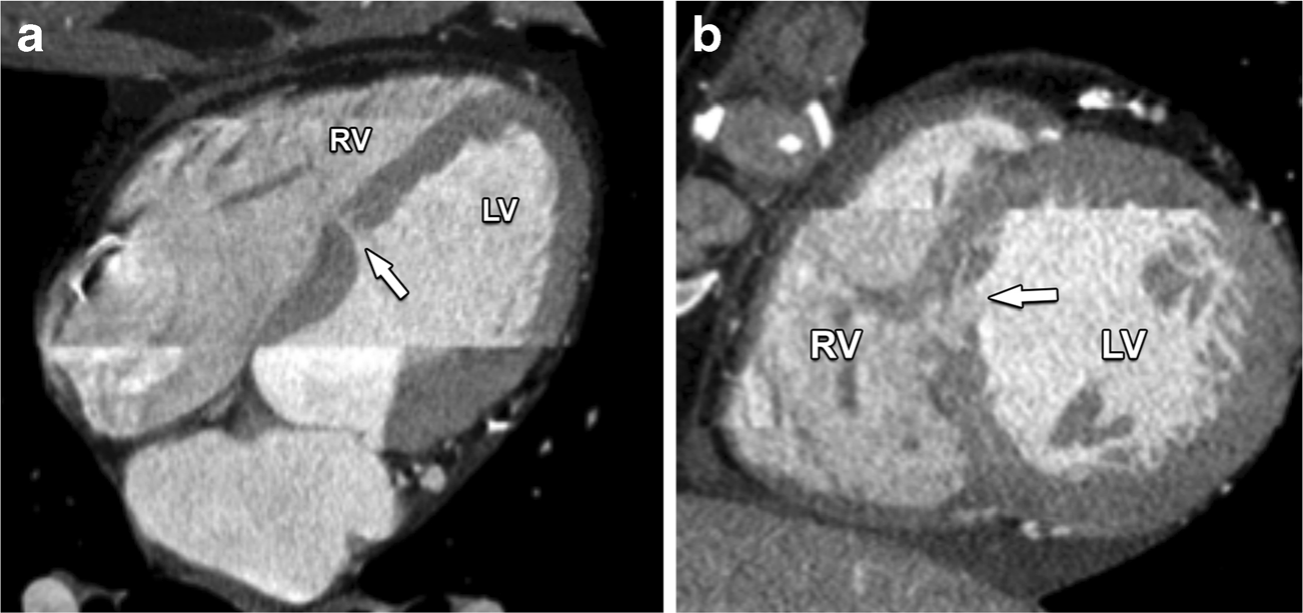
Four-chamber view (a) and two-chamber view (b) of contrast-enhanced CT in a patient with a muscular VSD. The defect (*arrow*) is confined to only the muscular part of the IVS. Like in membranous VSD, a large defect can lead to congestive heart failure or pulmonary vascular disease. *RV* right ventricle, *LV* left ventricle
